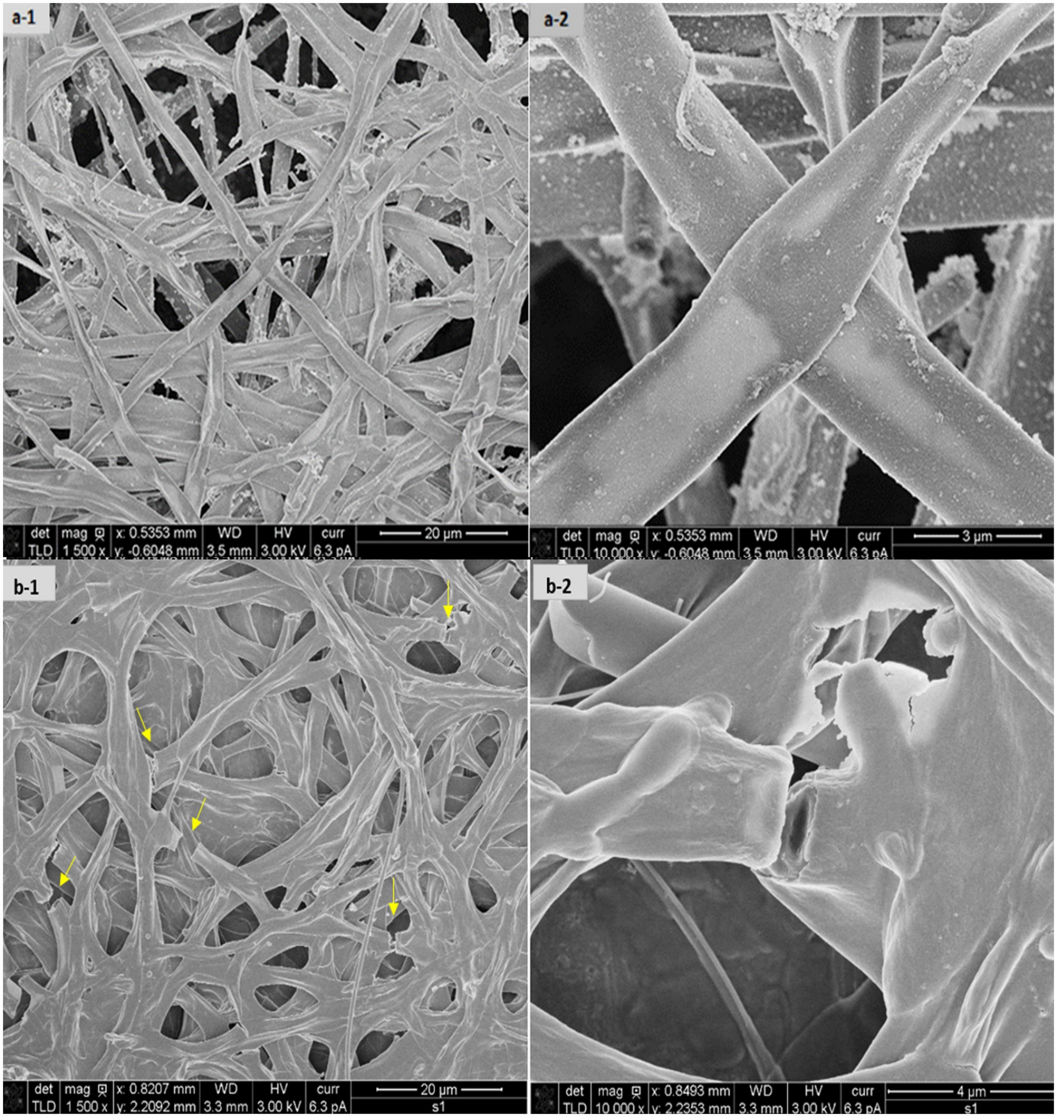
SEM images of (a) untreated and (b) ultrasound-treated (4 W) *F*. *oxysporum* biomass at different magnifications.
